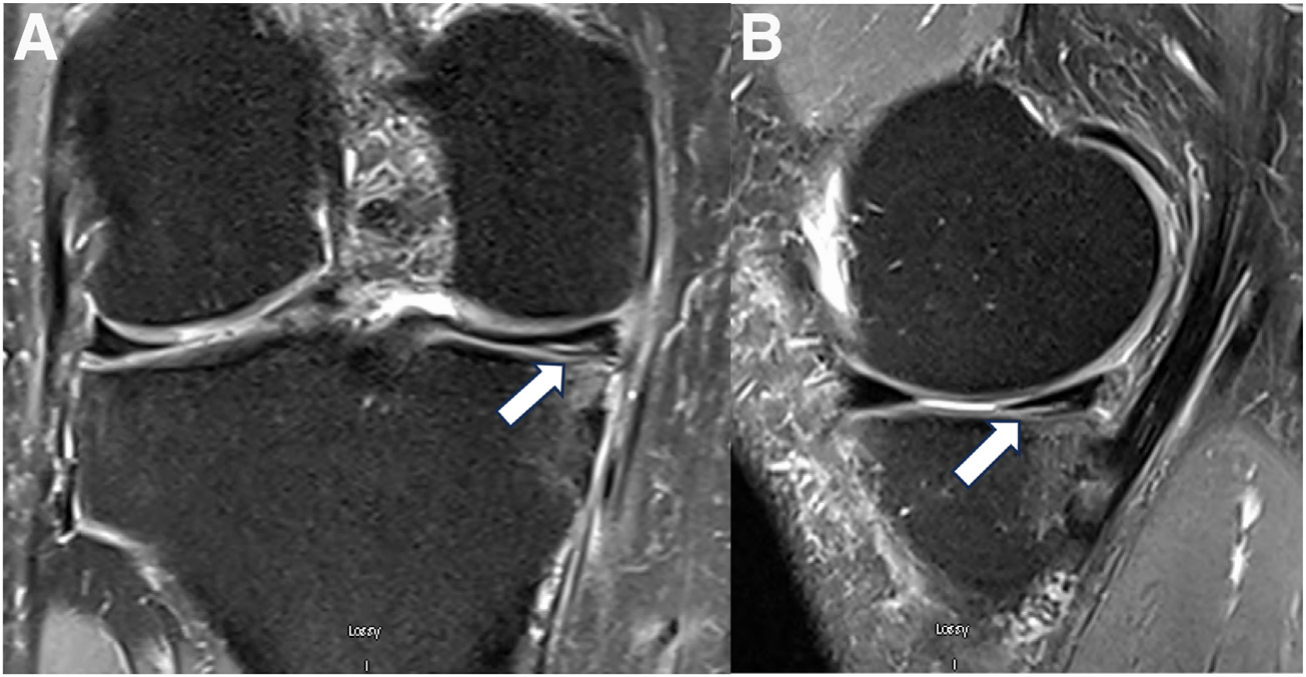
Degenerative grade 2 medial meniscal tear of the right knee in a 57-year-old man. The tear (white arrow) is located in the posterior horn of the medial meniscus on the coronal view (A) and sagittal view (B) of magnetic resonance imaging of the knee.
